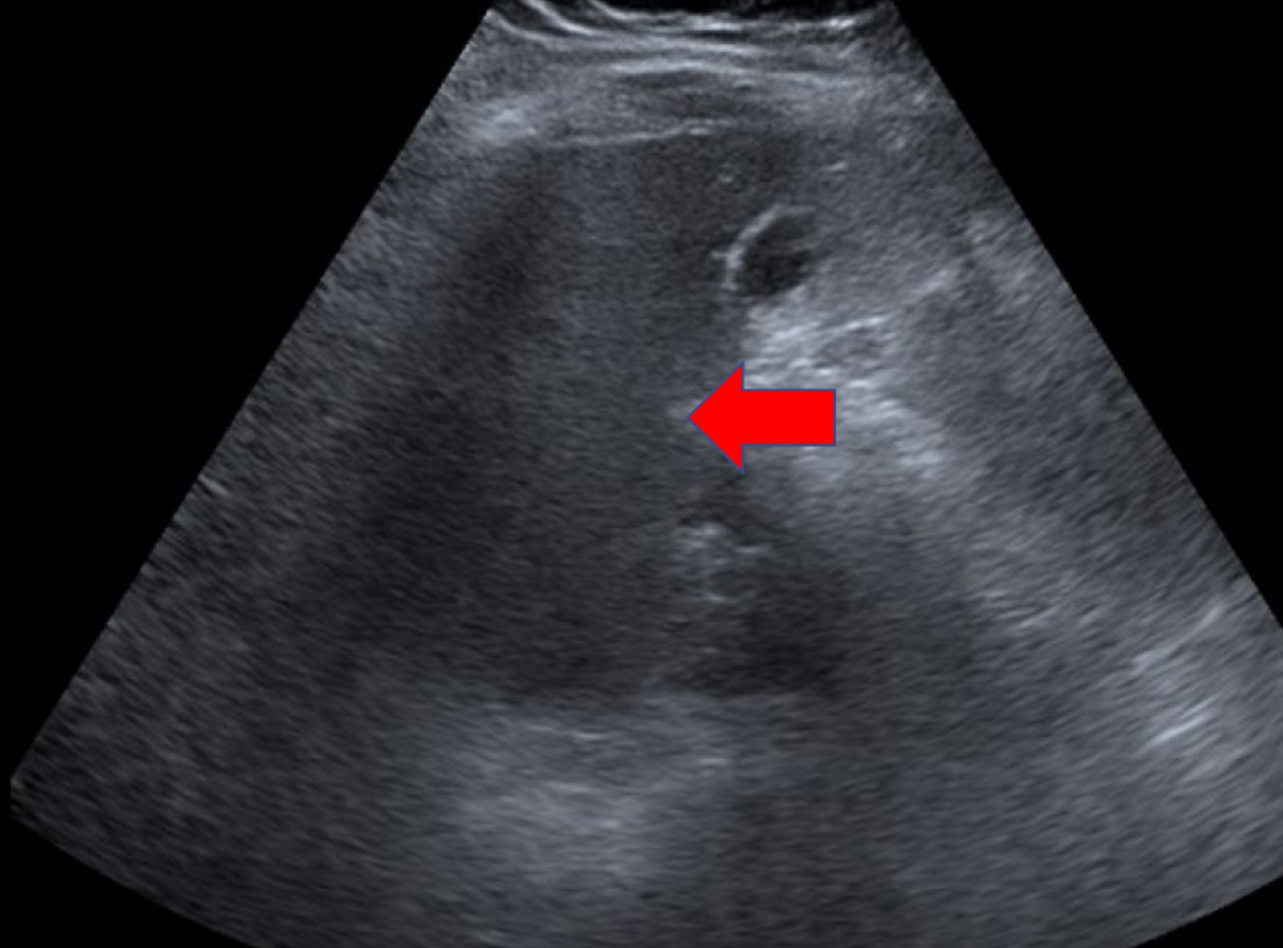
Ultrasonography showing a fluid collection in the liver
